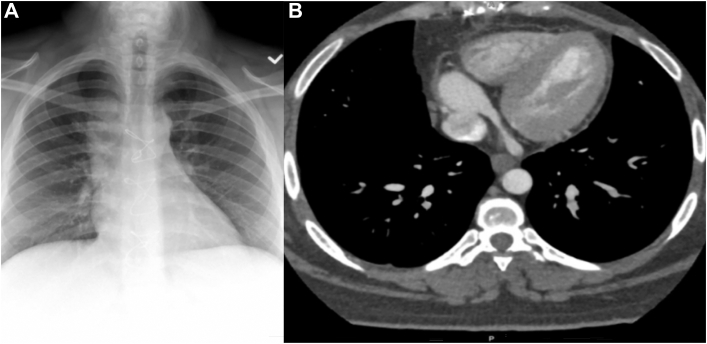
Postoperative imaging. (A) Posteroanterior chest roentgenogram showing reduced right-sided mediastinal shadow widening. (B) Axial computed tomographic angiography showing the resected azygos vein and a pericardial patch on the superior vena cava.
